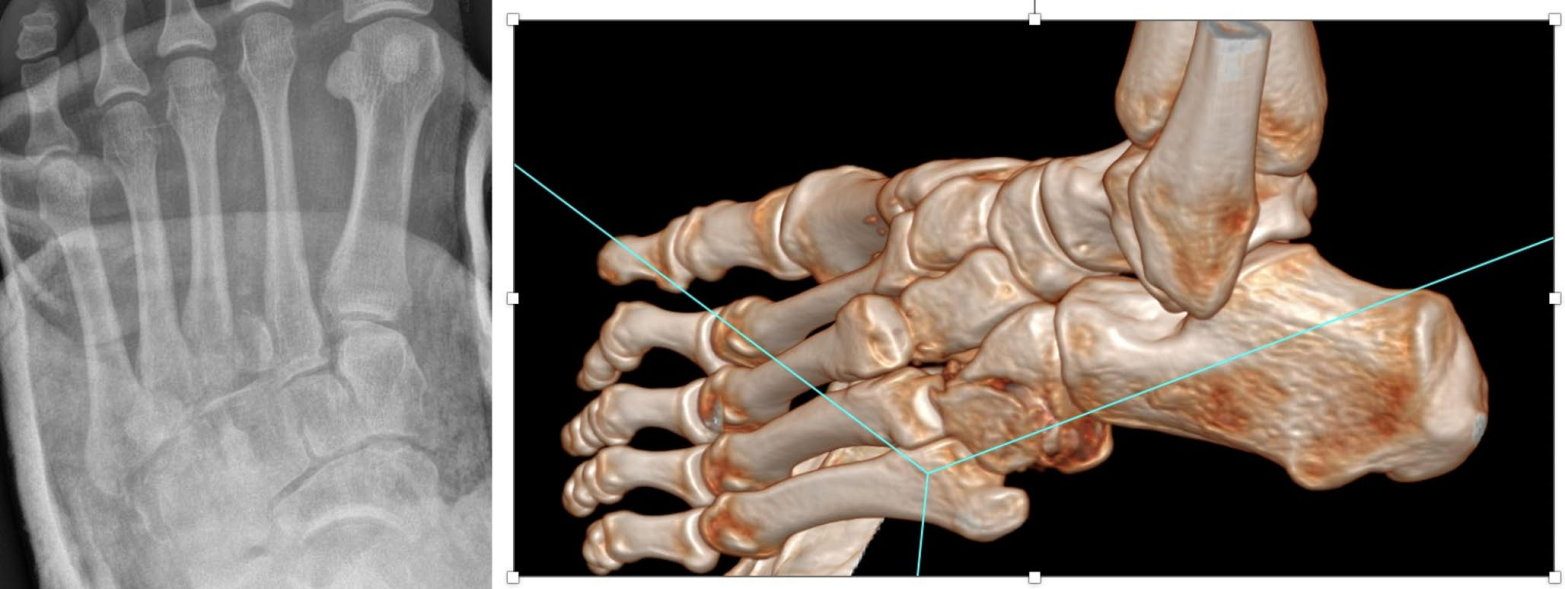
Radiograph and 3D surface rendering CT showing midfoot injury with affected central and lateral column in a polytrauma patient
